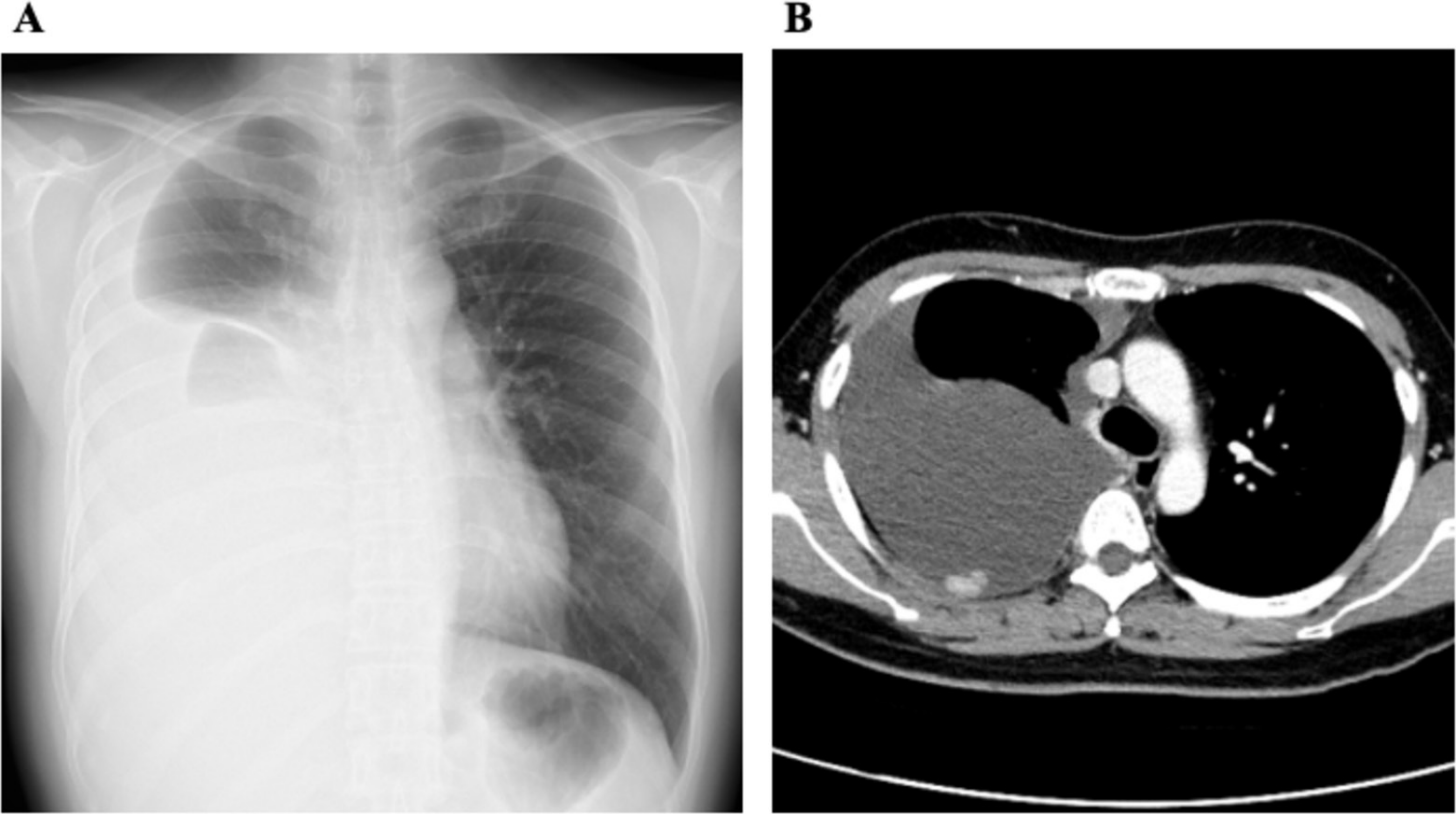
(A) Chest radiograph showing a large right‐sided pleural effusion. (B) Contrast‐enhanced chest CT demonstrating a massive right pleural effusion and multiple contrast‐enhancing nodular lesions along the pleural surface.
